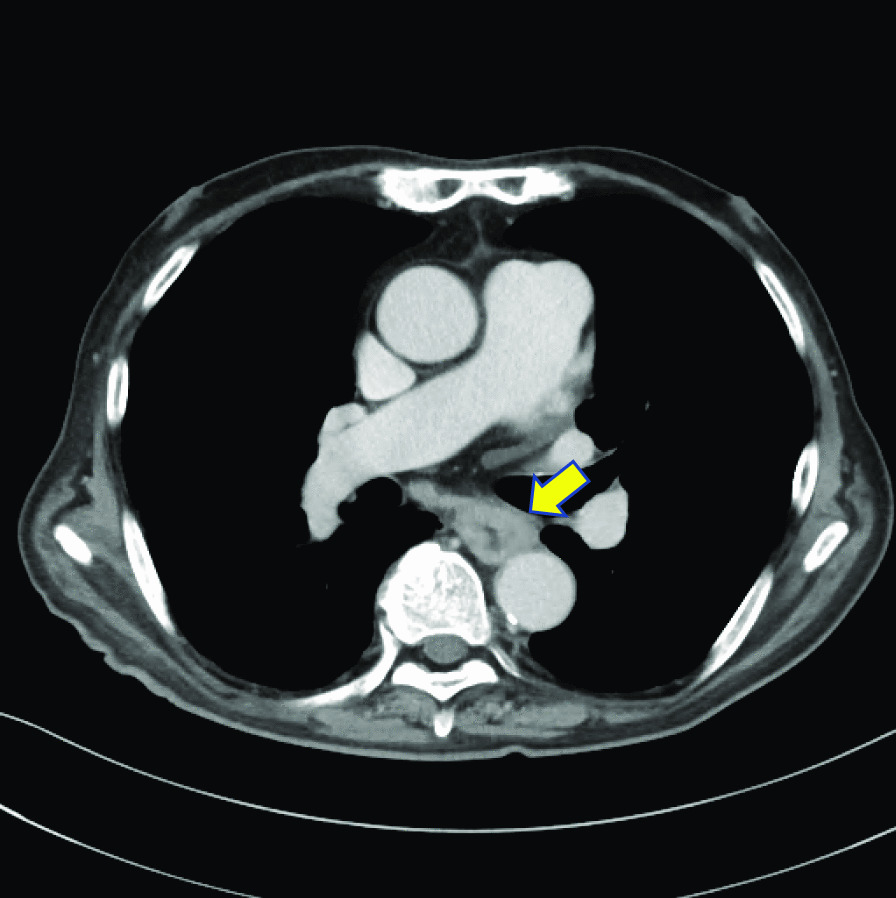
Enhanced CT examination demonstrating wall thickening with contrast effect in the middle thoracic esophagus (arrow)
